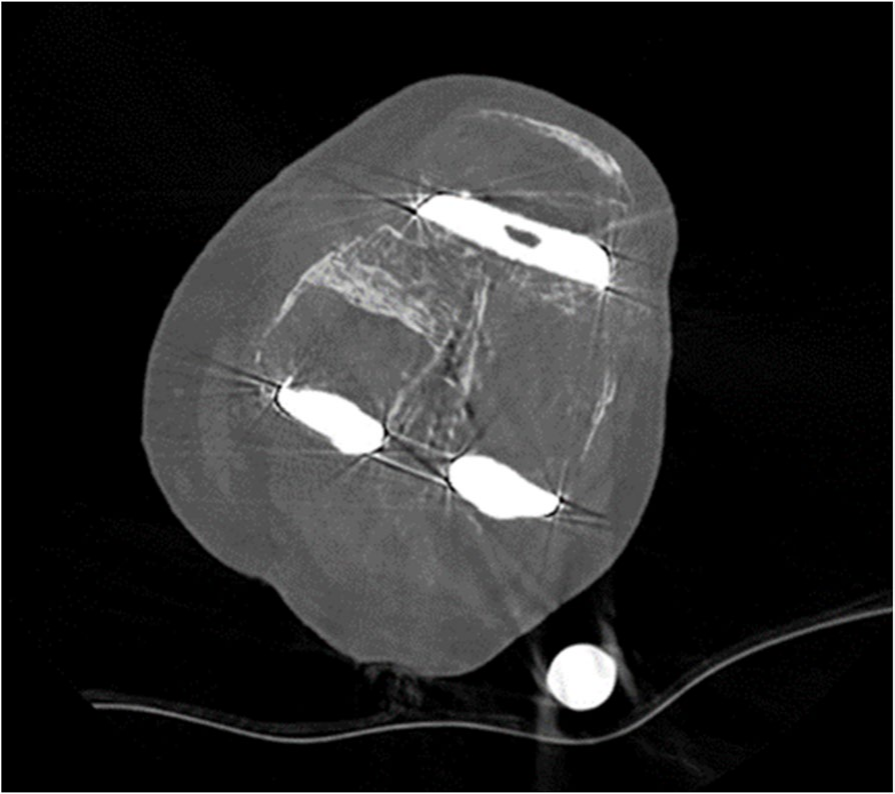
CT slice of the same level after MARS
